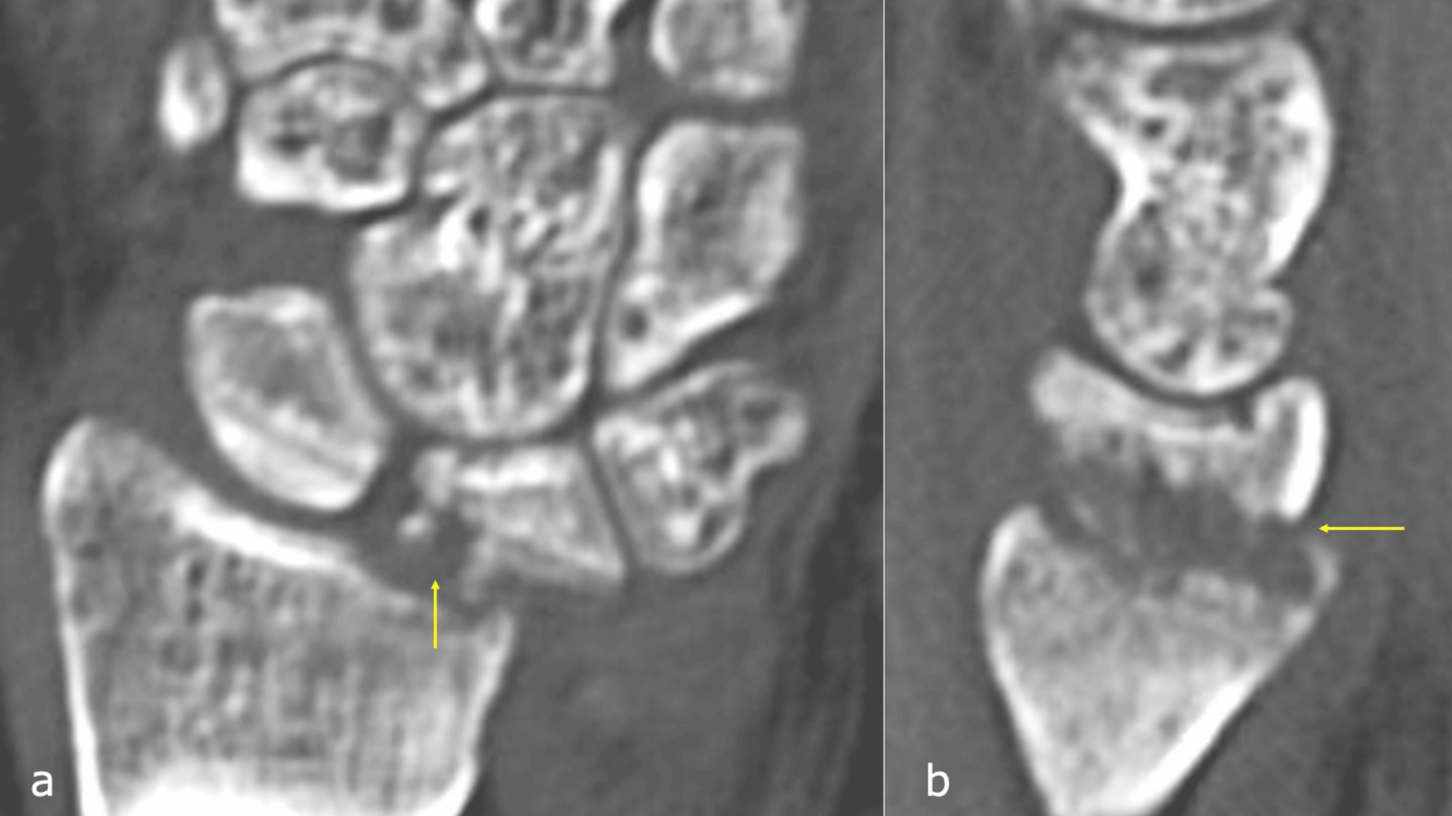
Computed tomography three weeks after Kirschner wire removal. a: Coronal view. b: Sagittal view. The lunate destruction and irregularity of the radial lunate fossa can be seen (arrow).
